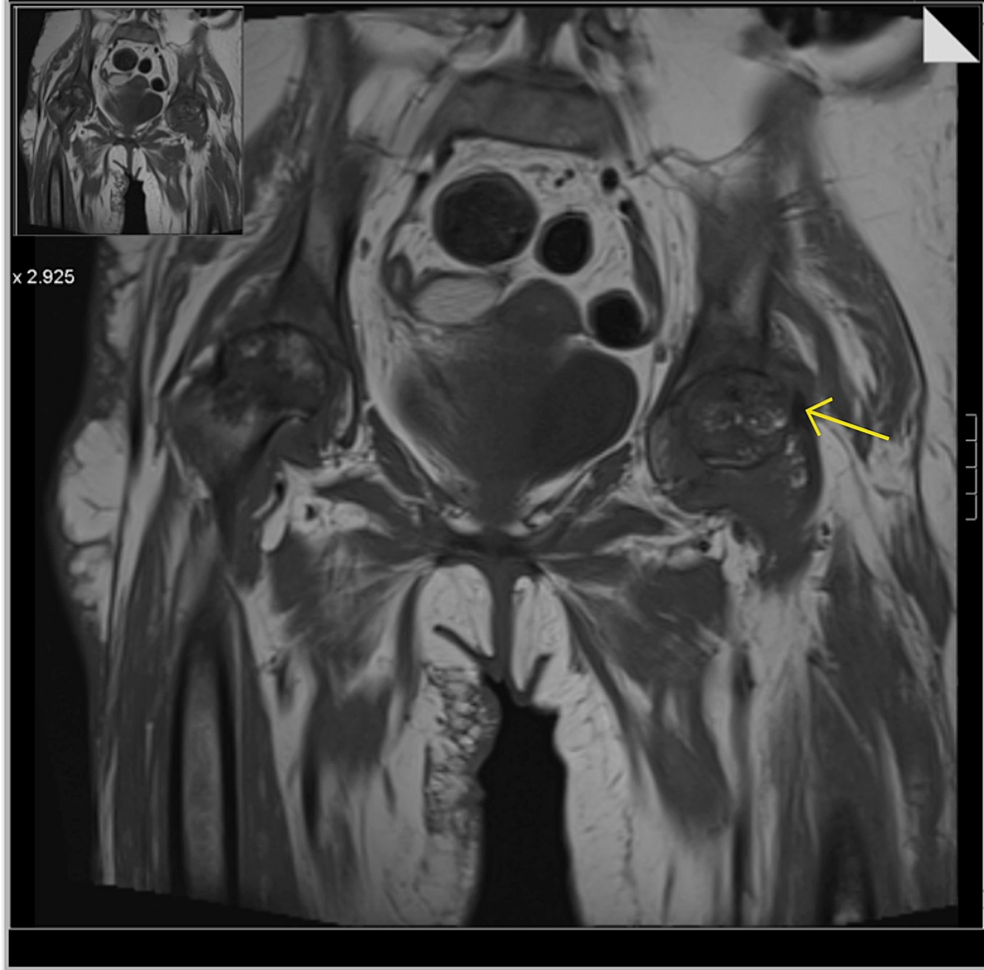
Pelvis MRI coronal view Yellow arrow shows the subchondral collapse in the superolateral aspect of the left femoral head
